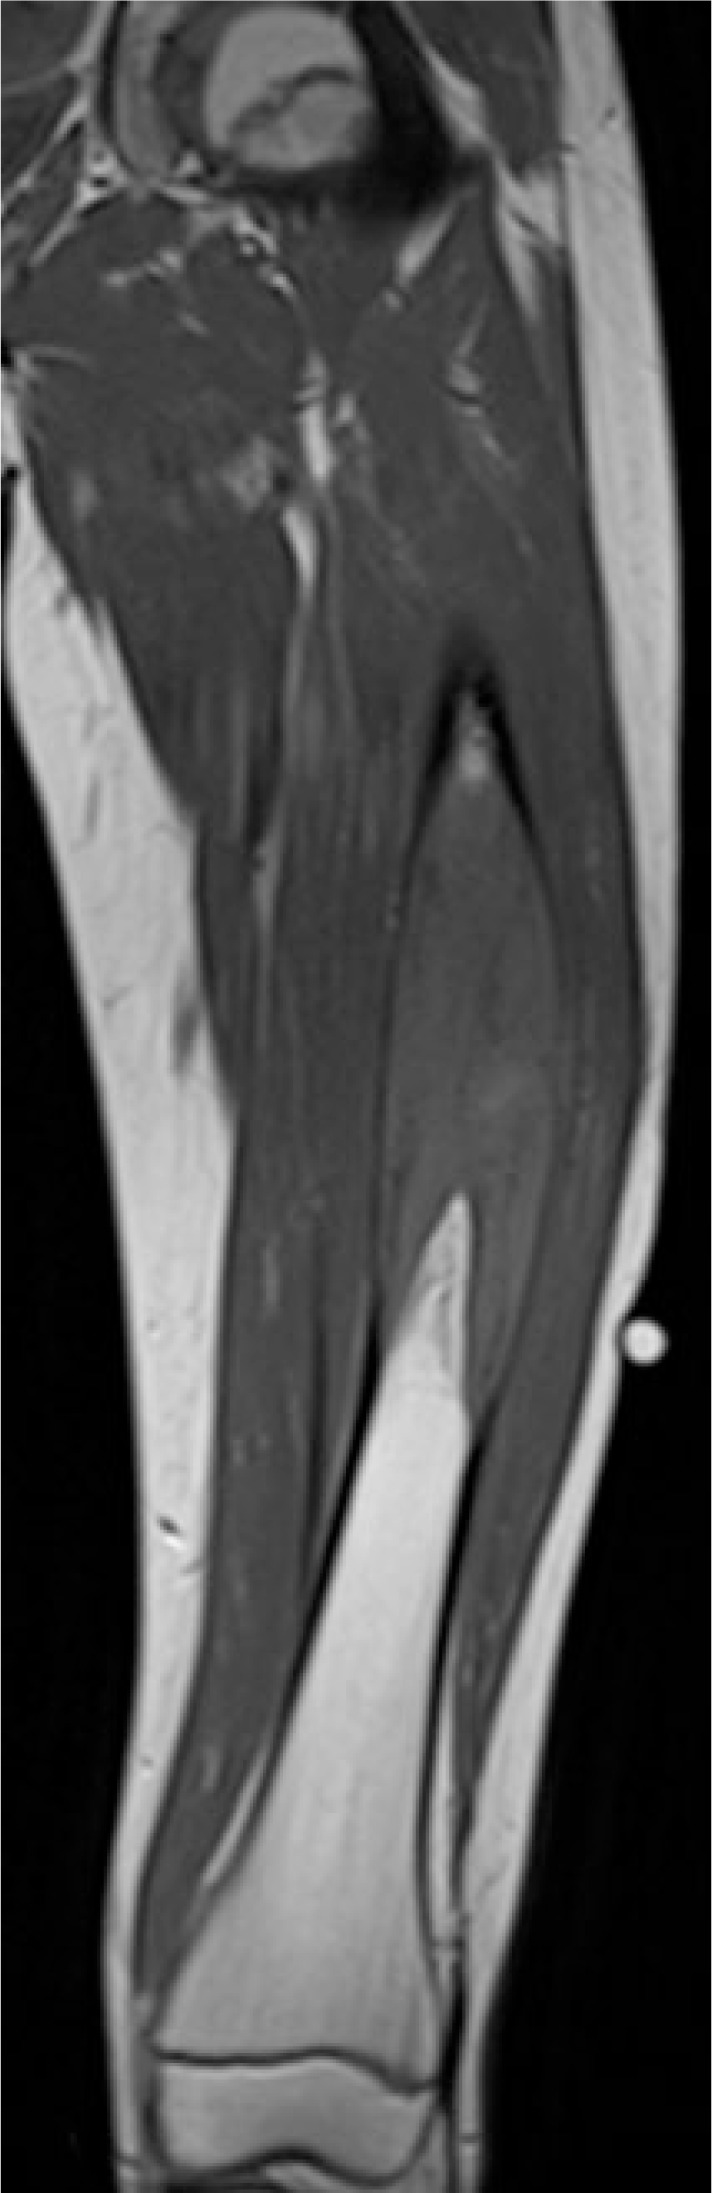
T1 weighed MR imaging detects a hyperintense, contrast medium enhanced, lesion in the mid of the left femur. Cortical destruction can be seen. The biopsy tract can be seen on the lateral side.
